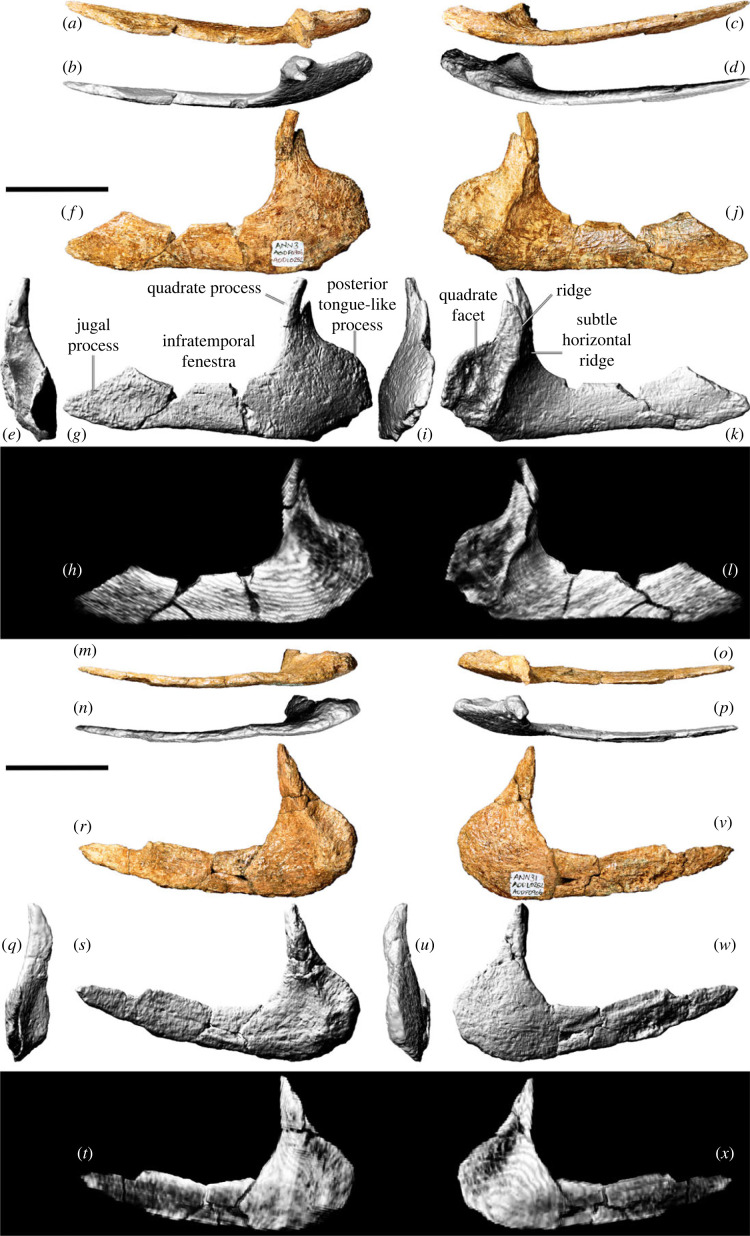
Diamantinasaurus matildae* referred quadratojugals (AODF 0906). (*a–l*) Left quadratojugal in (*a*,*b*) dorsal, (*c*,*d*) ventral, (*e*) anterior, (*f–h*) lateral, (*i*) posterior and (*j–l*) medial views. (*a*), (*c*), (*f*) and (*j*) are photographs, (*b*), (*d–e*), (*g*), (*i*) and (*k*) are three-dimensional models derived from surface scans, and (*h*) and (*l*) are three-dimensional models derived from CT data. (*m–x*) Right quadratojugal in (*m*,*n*) ventral, (*o*,*p*) dorsal, (*q*) anterior, (*r–t*) medial, (*u*) anterior, and (*v–x*) lateral views. (*m*), (*o*), (*r*) and (*v*) are photographs, (*n*), (*p–q*), (*s*), (*u*) and (*w*) are three-dimensional models derived from surface scans, and (*t*) and (*x*) are three-dimensional models derived from CT data. Scale bar = 50 mm.
